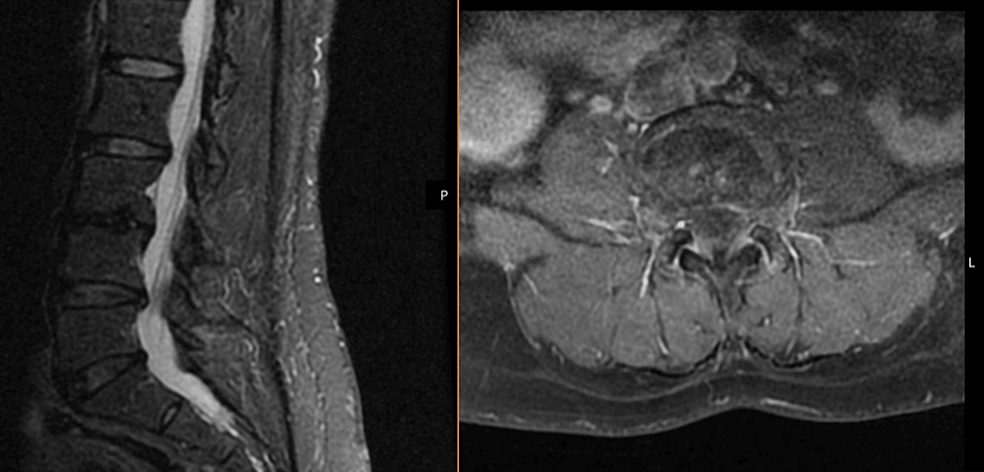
Inflammatory resolution in comparative lumbar magnetic resonance imaging after three months of treatment.
